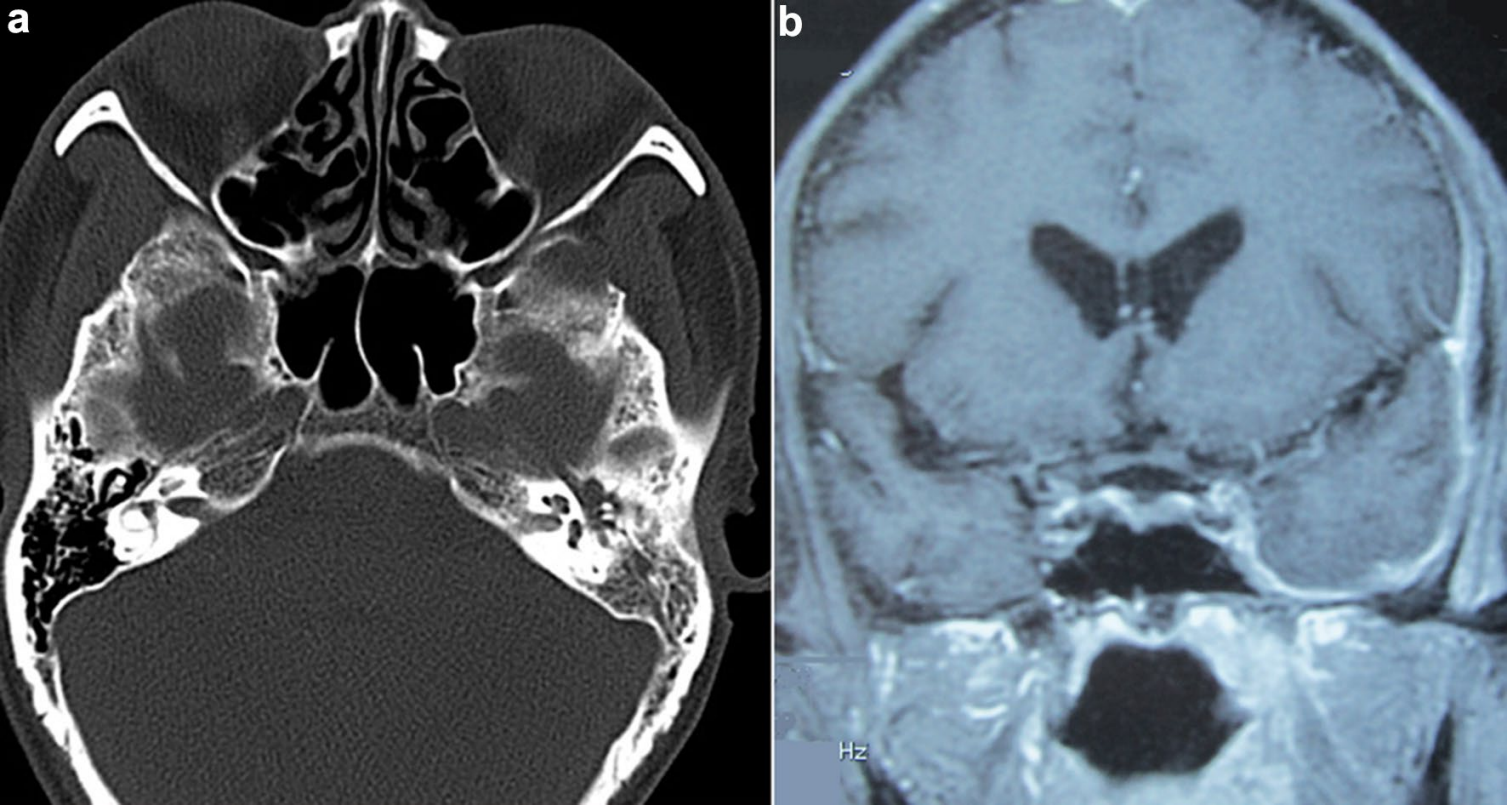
Imaging findings of a patient with ANCA-related HP and OM. a Axial CT: the left middle ear and mastoid cavities are filled with soft-tissue mass. b Coronal MRI: dural thickness of the left temporal lobe and tentorium cerebella
